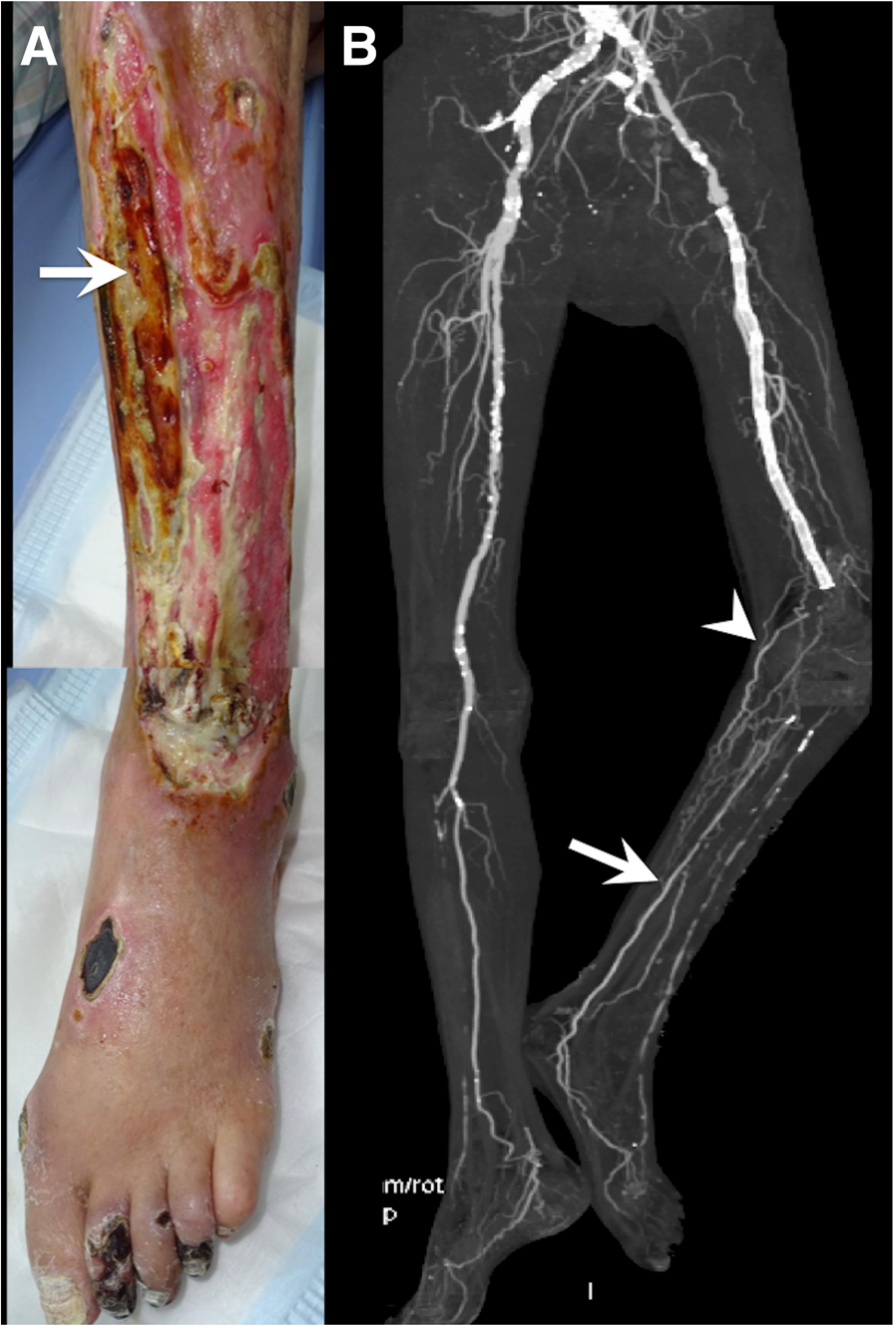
a Preoperative photograph showing the severe tissue defect at the lower leg in the form of an exposed tibia (arrow), multiple ulcers, and necrosis of the foot, including the toe, dorsum, lateral malleolus, and heel. b Preoperative computed tomography showed multiple arterial lesions, a patent posterior tibial artery (arrow), and well-developed sural artery (arrowhead)
